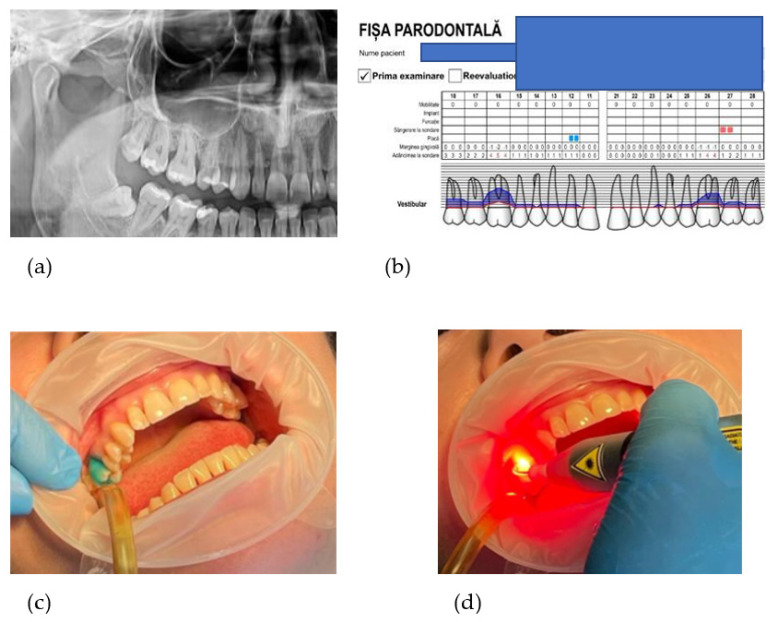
(a) patient X-ray showing the specific vertical bone loss in tooth 1.6; (b) periodontal chart showing the PD of the two selected sites for the study; (c) applying the photosensitizer dye liquid (Toluidine Blue Gel 0.005%; (d) photoactivation with the laser light at a wavelength optimized for toluidine blue solution (635 nm).
